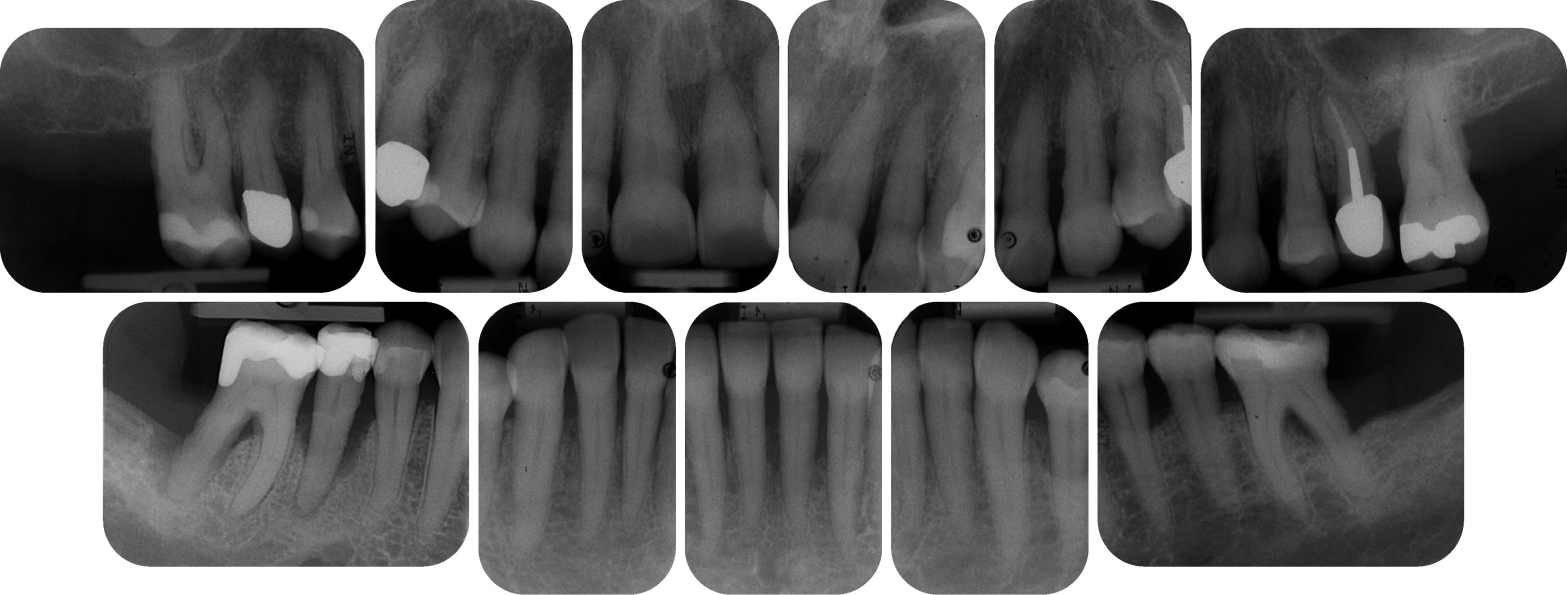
Radiographic view at baseline (ie, before steps 1 and 2 of periodontal therapy) depicting severe horizontal and vertical bone loss
